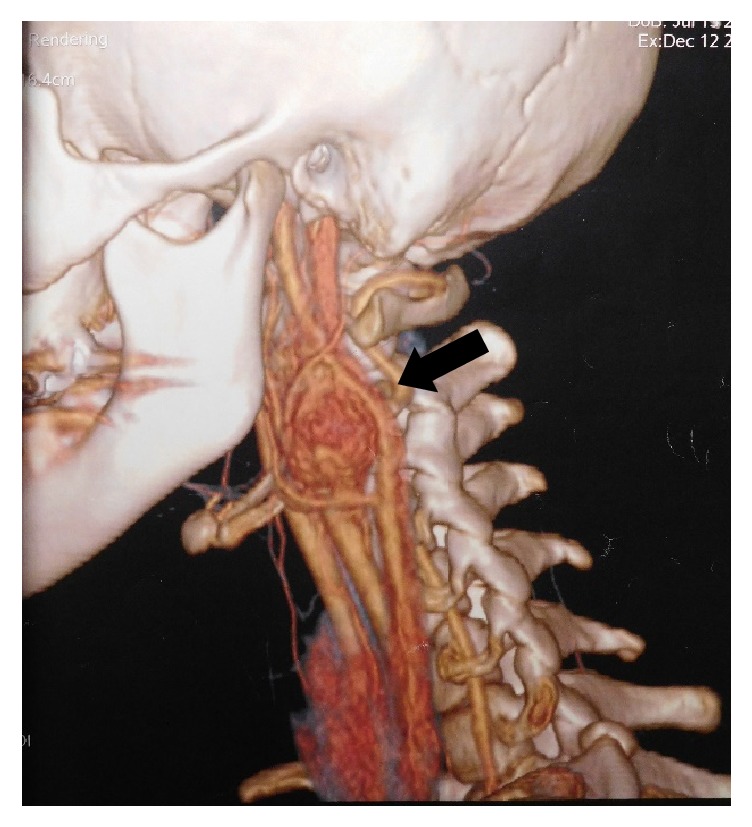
3-dimensional (3D) cervical-computed tomography angiography (CTA), lyre sign (arrow).
